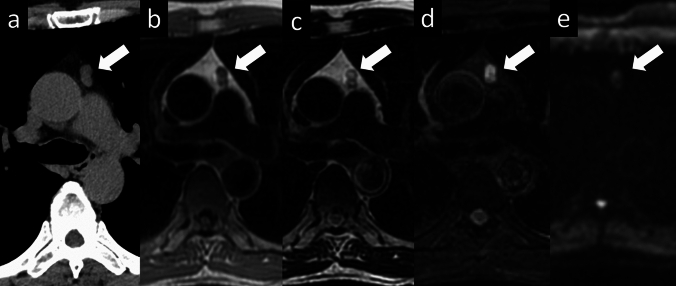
Thymoma (type A). A 73-year-old man with type A thymoma. a Chest CT scan shows a 1.3-cm, slightly lobulated, prevascular nodule (arrow). b, c MRI shows a prevascular nodule with low signal intensity on T1WI b and high signal intensity on T2WI c (arrow). d MRI shows high intensity on fat-suppressed T2WI (arrow). e MRI shows slightly high signal intensity on DWI (arrow) and a high ADC value (3.4 × 10^–3^ mm^2^/s). The pathological diagnosis was type A thymoma. CT, computed tomography; MRI, magnetic resonance imaging; T1WI, T1-weighted image; T2WI, T2-weighted image; DWI, diffusion-weighted imaging; ADC, apparent diffusion coefficient
